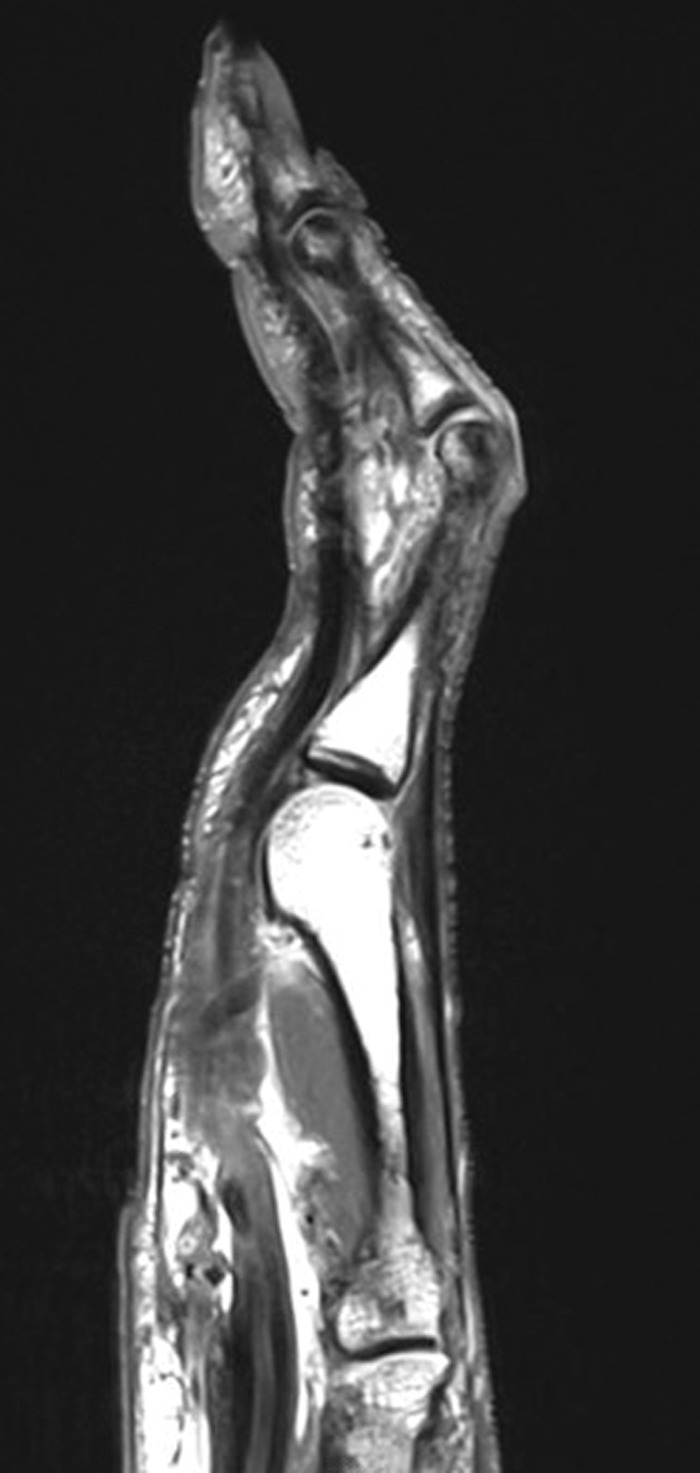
Sagittal proton density magnetic resonance imaging demonstrates complete ruptures of the annular 2 (A2) pulley and flexor digitorum superficialis tendon of the long finger. Bowstringing of the flexor digitorum profundus tendon is suggestive of concomitant annular 3 (A3) pulley rupture.
